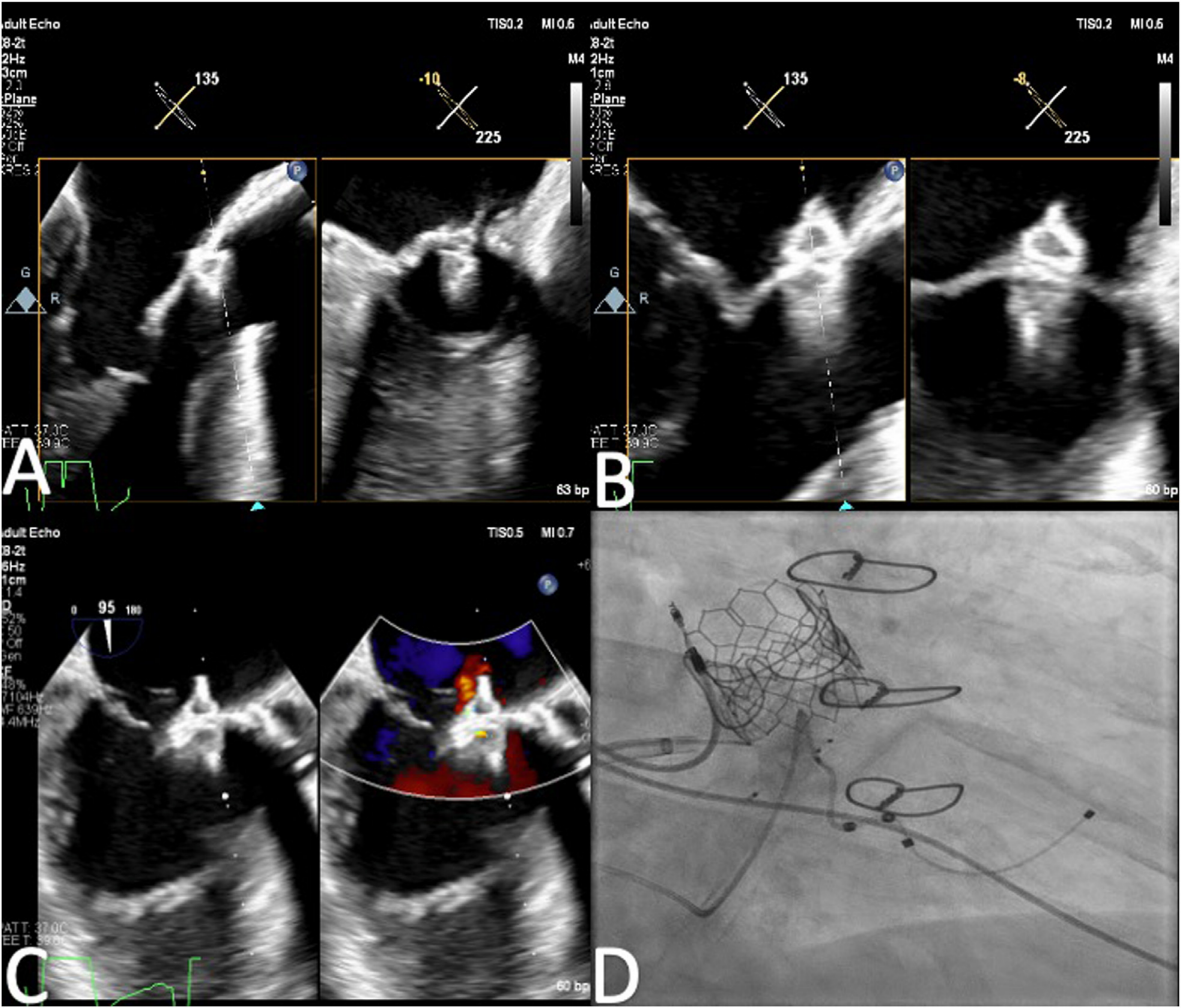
(A) Transesophageal echocardiogram biplane view of the first Amplatzer Vascular Plug 4 (AVP 4) being deployed. (B) First AVP 4 deployed on the atrial and ventricular sides of the anterior mitral leaflet. (C) The second AVP 4 is placed due to moderate residual mitral regurgitation, demonstrating appropriate placement of the second AVP 4 with trace residual mitral regurgitation. (D) Fluoroscopic view showing both AVP4.
